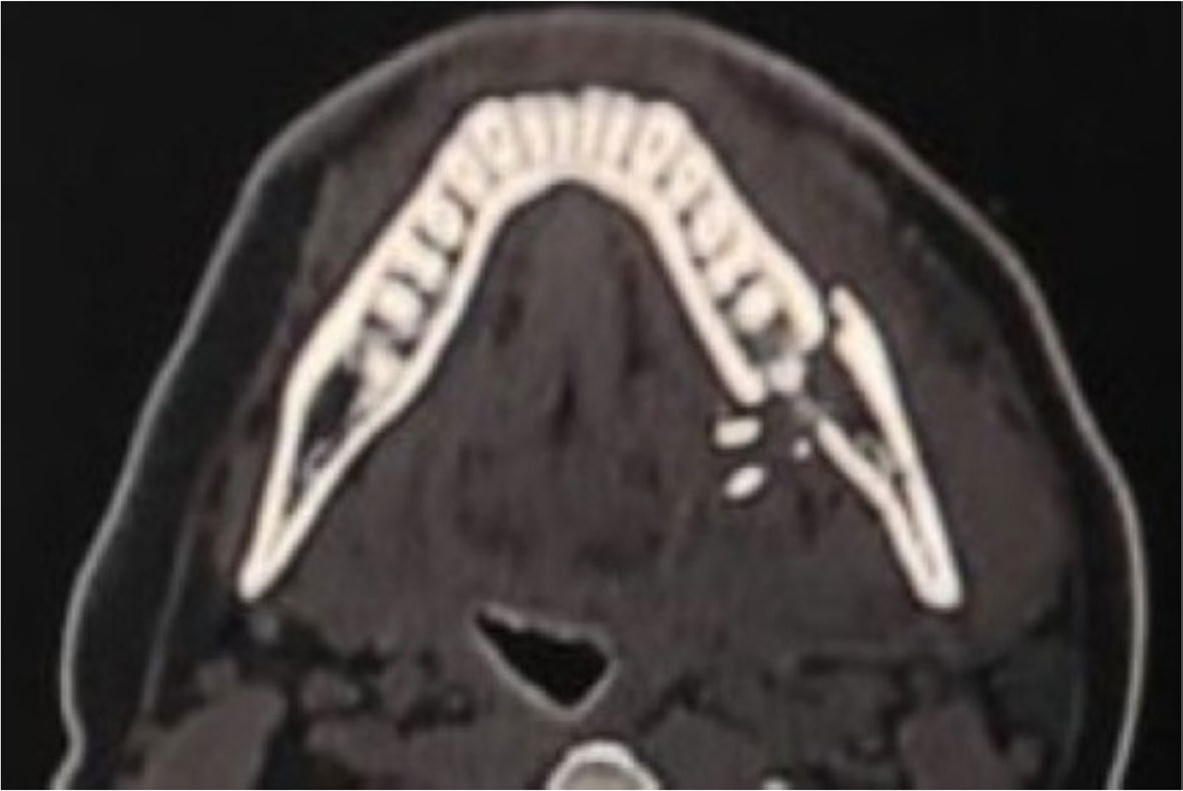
Preoperative axial view of CT-scan showing the fracture line
